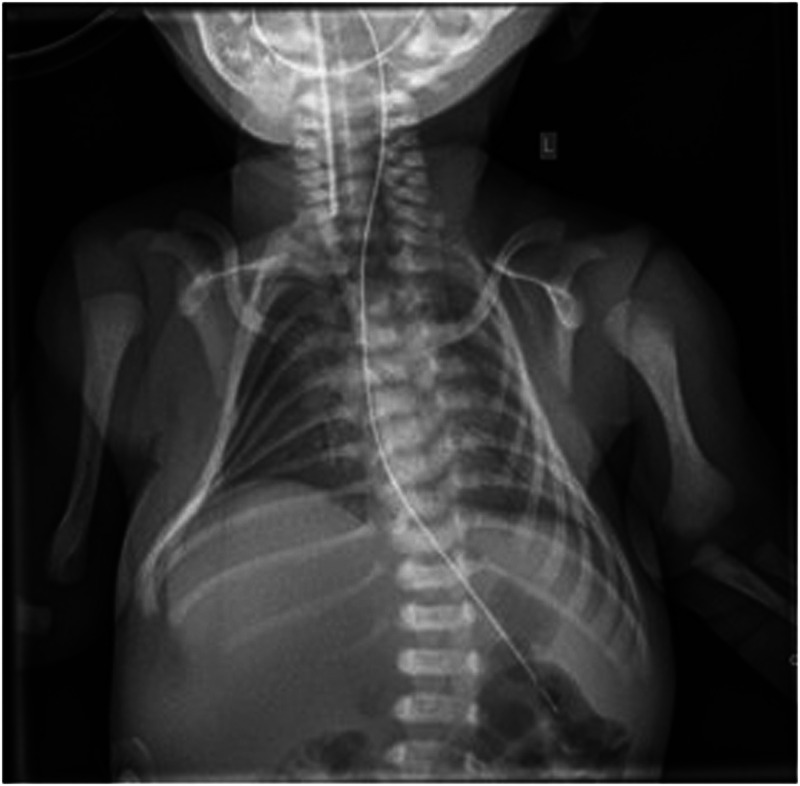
Chest x-ray on first day of life showing severe pulmonary hypoplasia with bell-shaped thorax.
